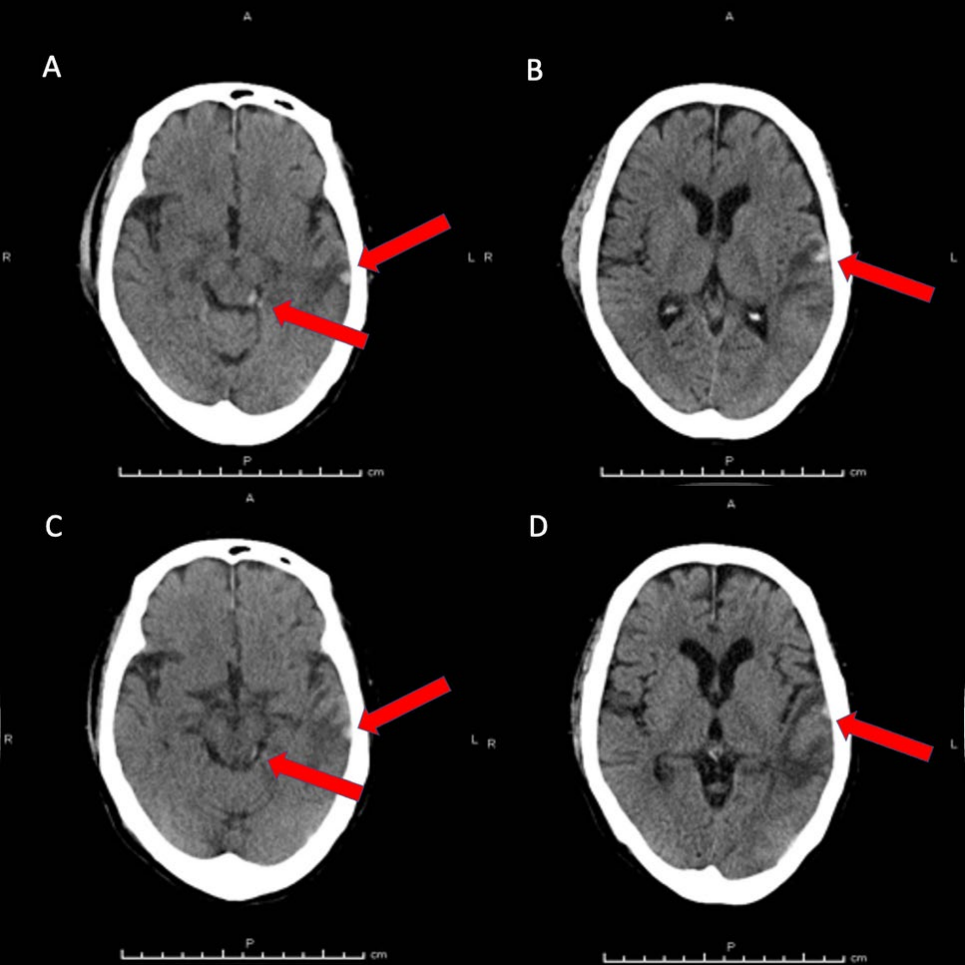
Axial CT on hospital day 2 (A and B) and on hospital day 7 (C and D). No enlargement of hemorrhages is evident. Red arrows show the respective lesions
